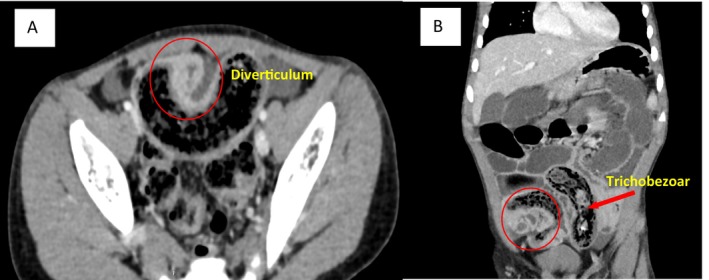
Computed tomography (CT) axial (A) and coronal (B) images demonstrating small‐bowel obstruction with dense matter accumulation in the terminal ileum (red arrow), and additional findings suggesting a complicated Meckel's diverticulum (red circle).
